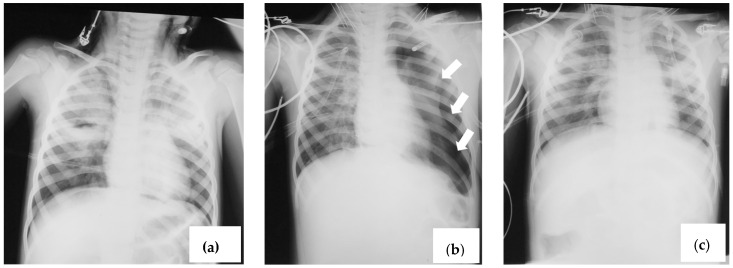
Thoracic X-ray (anterior–posterior) showing (a) diffuse interstitial infiltrate, consolidations, cavitation (right), and small pneumothorax; (b) large left pneumothorax indicated with arrows; (c) lung re-expansion following pleurotomy with exsufflation.
